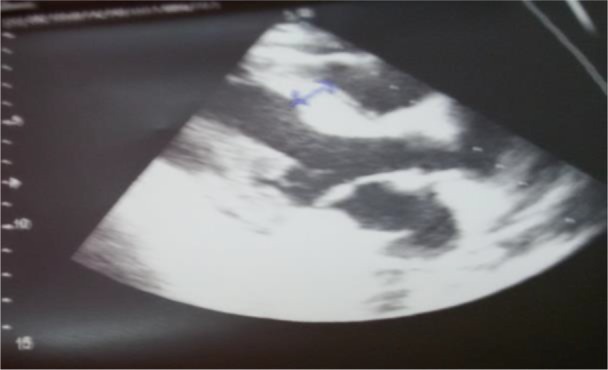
Echocardiography image of patient.
